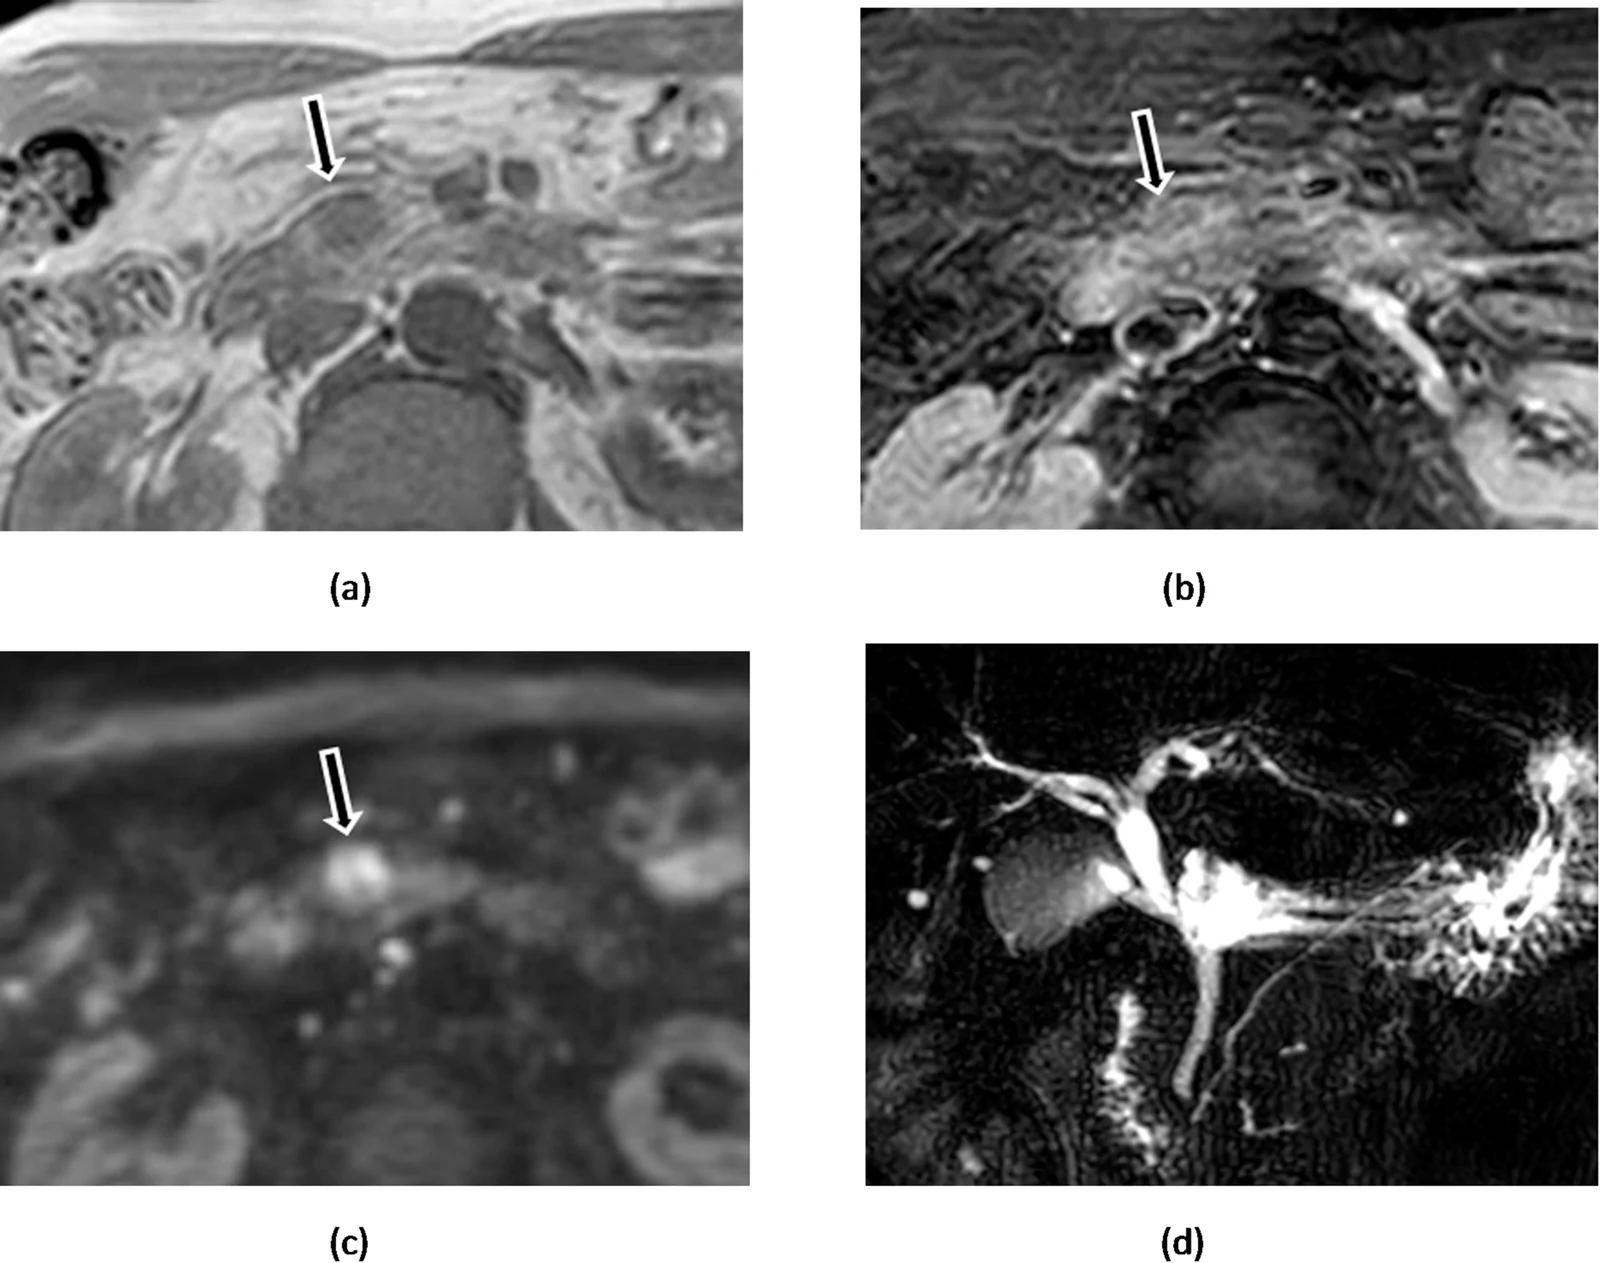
A 72-year-old male patient with pancreatic ductal adenocarcinoma in the pancreatic head measuring 14 mm. T1-weighted (a) and diffusion-weighted (c) images demonstrate a well-demarcated tumor (arrows) in the pancreatic head. The tumor is undetectable on fat-saturated T2-weighted imaging (b). No stenosis or dilatation of the main pancreatic duct distal to the tumor is observed on magnetic resonance cholangiopancreatography (d)
